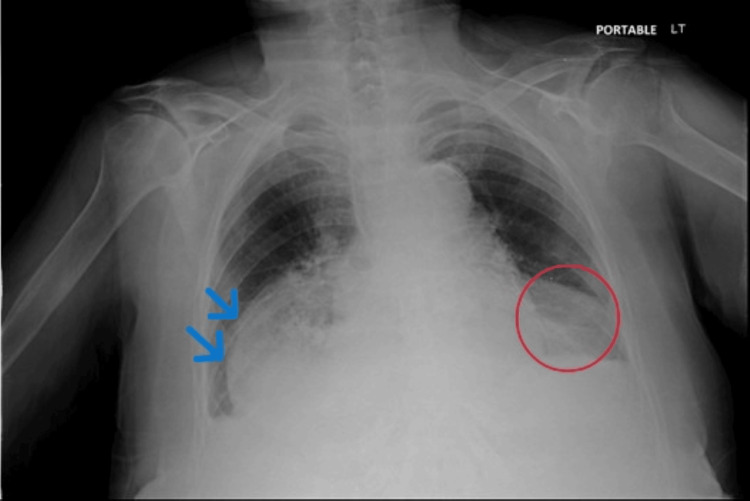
Chest X-ray demonstrated a large retrocardiac air-fluid level consistent with a hiatal hernia (blue arrows), along with a mildly right-shifted cardiac silhouette (red circle).
